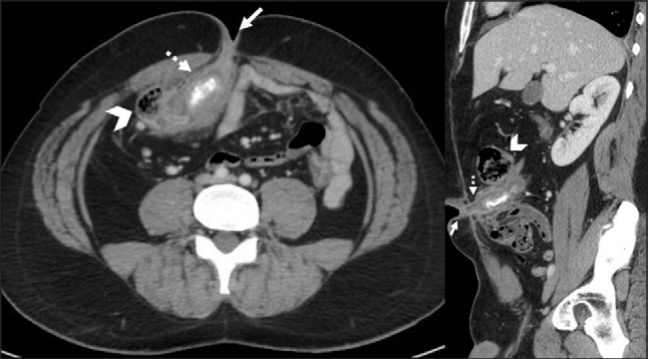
Coronal reformatted and axial contrast medium-enhanced CT-scan images showing dilated and thickened intestinal structure (dotted arrow) between the caecum (arrowhead) and the umbilicus (arrow), containing a calcified deposit.
